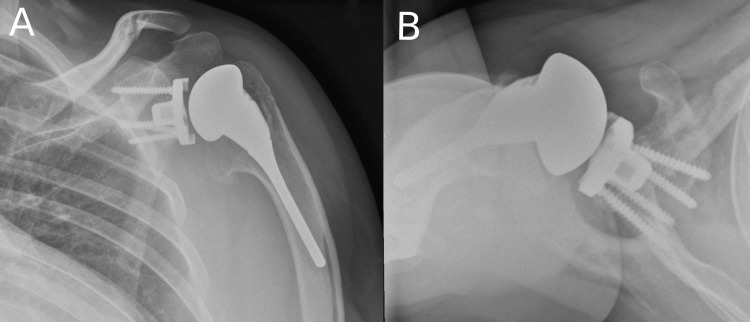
X-rays of the left shoulder immediately after the index operation. (A) Anteroposterior view; (B) Axial view
